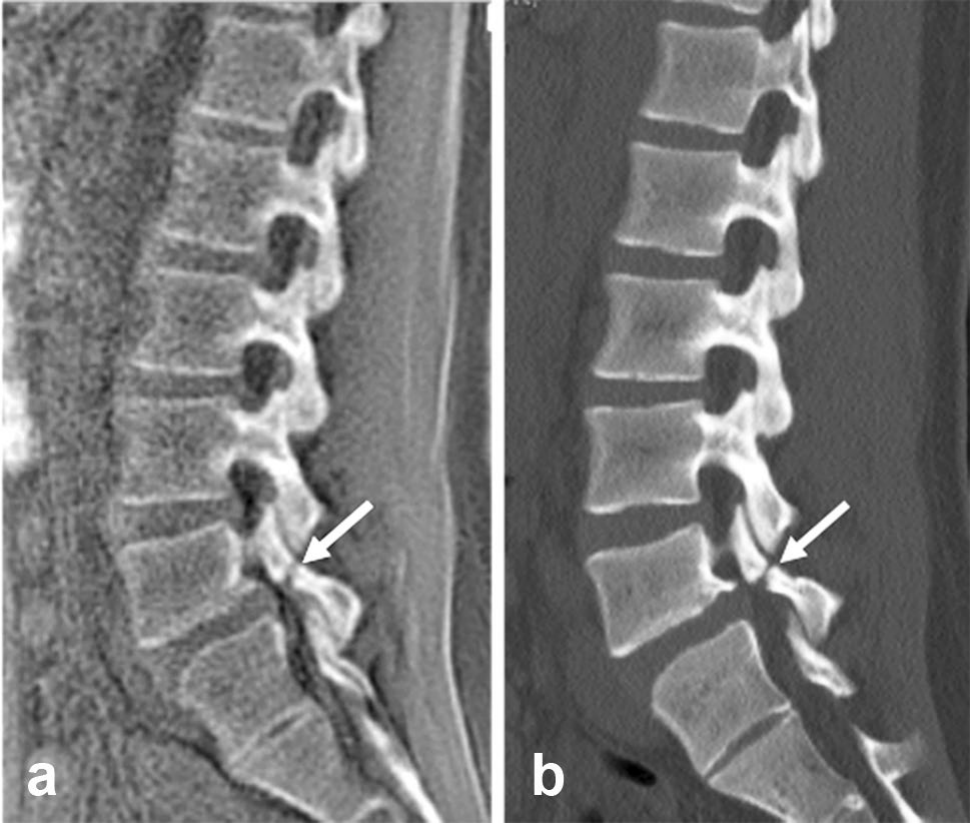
Spondylolysis. Spondylolysis is a defect in the pars interarticularis of the neural arch often seen in the adolescent population. Sagittal MR bone image a obtained with the ZTE sequence at 1.5 T shows a defect in the pars interarticularis of L5 in a 19-year-old female patient (arrow). Sagittal CT bone image b shows the same finding (arrow). (Courtesy of Akira Fujikawa, MD, PhD, Self-Defense Forces Central Hospital, Tokyo, Japan)
